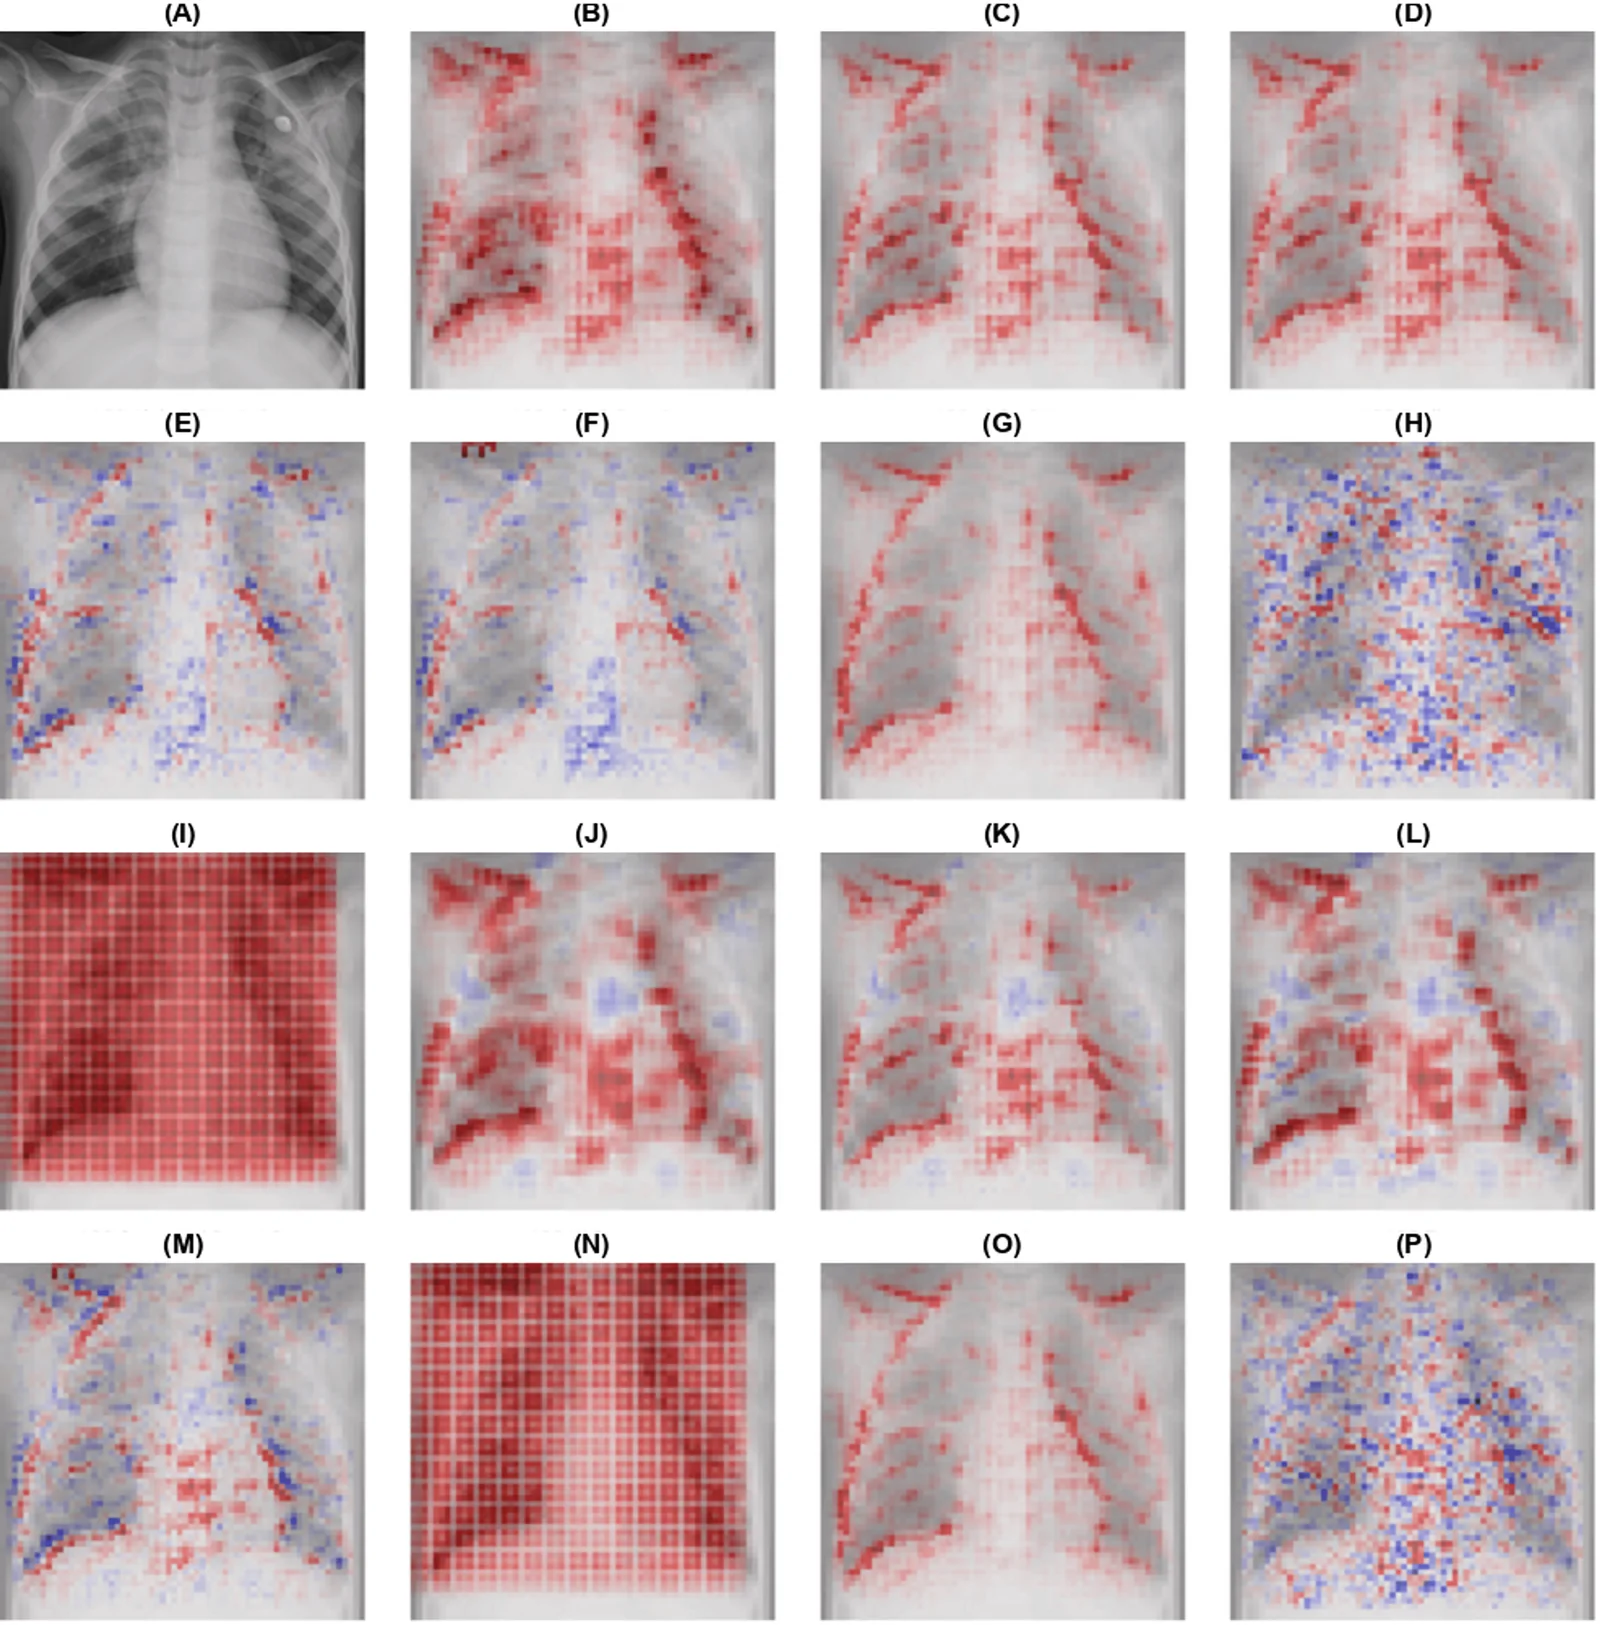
Representative CWP chest X-ray analyzed with Deep Taylor and LRP rules for the 3-class model. Panels show the original image and corresponding relevance maps for each method. (A) Original chest X-ray; (B) Deep Taylor Bounded; (C) Deep Taylor; (D) LRP Alpha 1 Beta 0; (E) LRP Alpha 2 Beta 1 IB; (F) LRP Alpha 2 Beta 1; (G) LRP Alpha Beta; (H) LRP Epsilon; (I) LRP Flat; (J) LRP Sequential Preset A Flat; (K) LRP Sequential Preset A; (L) LRP Sequential Preset B Flat; (M) LRP Sequential Preset B; (N) LRP W Square; (O) LRP Z Plus; (P) LRP Z. COVID-19: coronavirus disease 2019; CWP: COVID-19 with pneumonia; LRP: layer-wise relevance propagation; IB: input-layer bounded. Panel (A) was adapted from the Covid_w/wo_pneumonia chest Xray dataset [19] and is used under the Community Data License Agreement-Sharing 1.0 (CDLA-Sharing-1.0); all relevance maps and annotations were generated by the authors.
